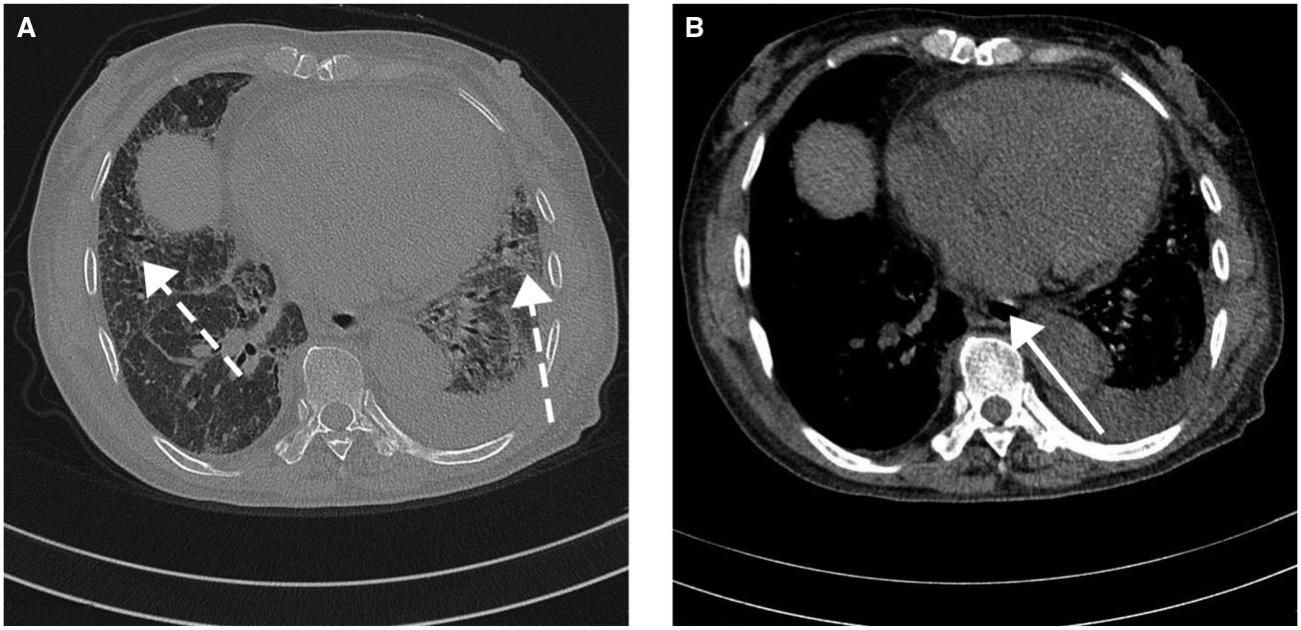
High-resolution CT (HRCT) of the chest. Chest HRCT images showed subpleural ground-glass shadows and grid-like lesions compatible with ILD. Oesophageal dilation, traction bronchiectasis, bilateral pleural effusion and a small amount of pericardial effusion were present. (A) Chest HRCT, pulmonary window. White broken arrows indicate ILD. (B) Chest HRCT, mediastinal window. White arrow indicates oesophageal dilation
